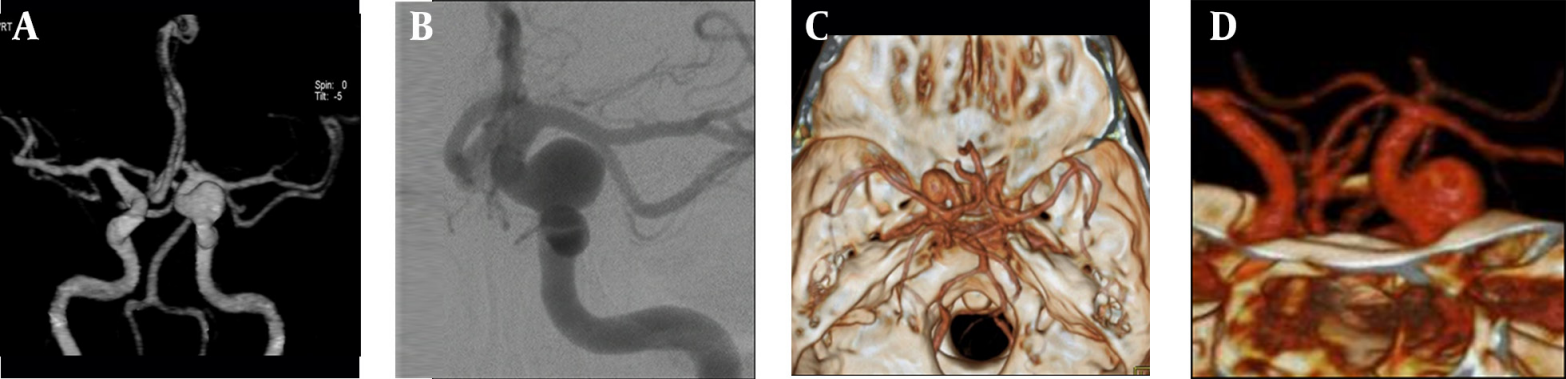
A 55-year-old woman with a left ICA aneurysm A) BSCTA anteroposterior view; B) DSA lateral view; C) CTA superoinferior view; D) CTA lateral view. The aneurysm located in the left ICA was partially detected in CTA, and very clearly in BSCTA.
